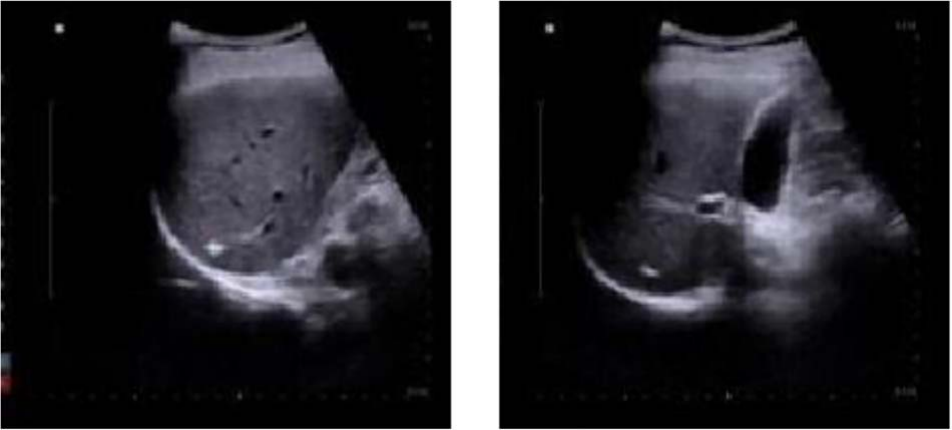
Abdominal color ultrasound findings on the 30th day after surgery.
